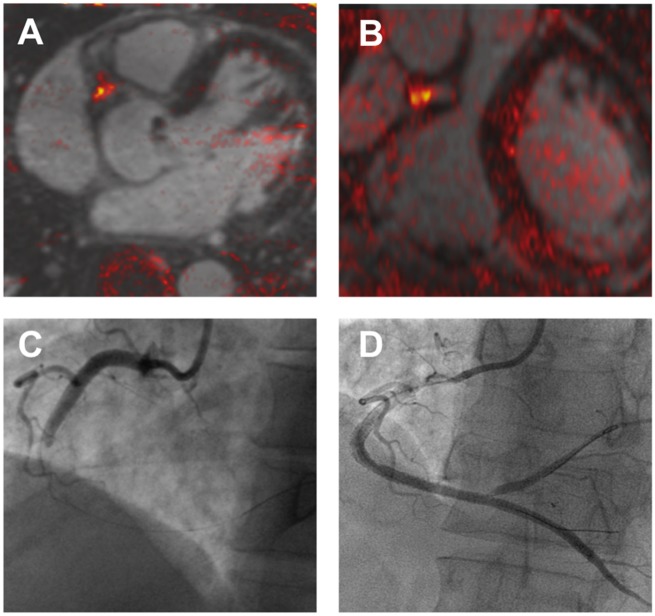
Coronary atherosclerosis T_1_-weighted characterization with integrated anatomical reference (CATCH). T_1_-weighted magnetic resonance coronary angiogram of a patient who presented with an inferior myocardial infarction shows evidence of a focal high intensity lesion (arrows) in the right coronary artery on magnetic resonance imaging (*A* and *B*). Subsequent coronary angiogram demonstrated occlusion of the mid-right coronary artery (*C*) with restoration of flow following thrombus aspiration (*D*).
